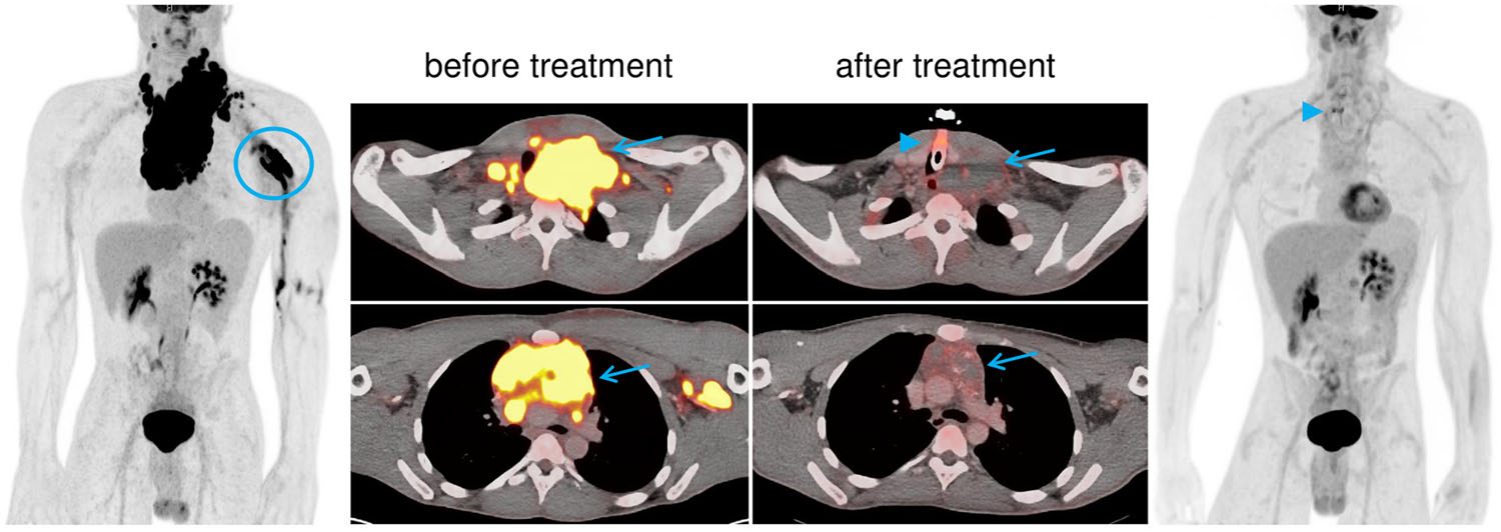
A 27-year-old male patient with diffuse large B-cell lymphoma. Before treatment (baseline), a markedly hypermetabolic nodal conglomerate extending from the anterior mediastinum to the neck is visible (blue arrows), and additional hypermetabolic neck nodes are also present (stage II). After treatment, the mass has markedly decreased, now includes necrotic components and calcifications, and shows residual uptake ≤ liver uptake, corresponding to a 5-PS (Deauville) score of 3 and consistent with complete (metabolic) response. The hypermetabolic mediastinal focus on PET after treatment (arrowheads) is related to a new tracheostoma. The marked, left axillary uptake on the maximum intensity projection before treatment is due to venous congestion and slow blood flow secondary to the mediastinal mass, with FDG accumulation in the axillary vessels (blue circle)
